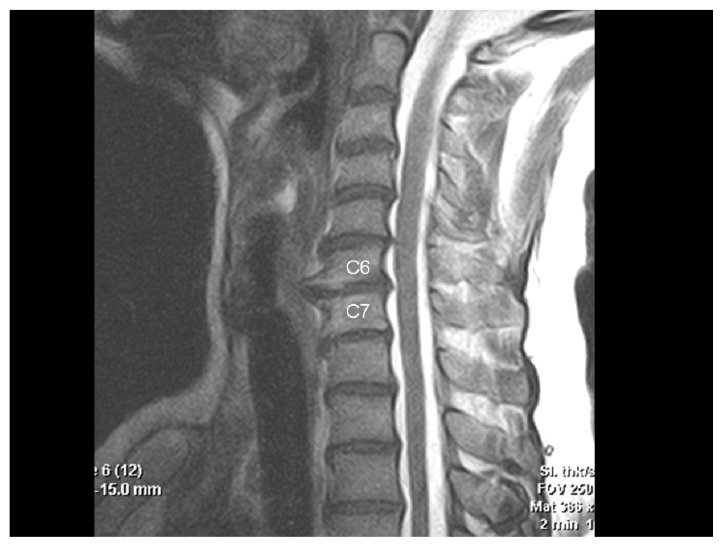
Sagittal T2-w MRI demonstrates compression of the esophagus by anterior osteophytes.
